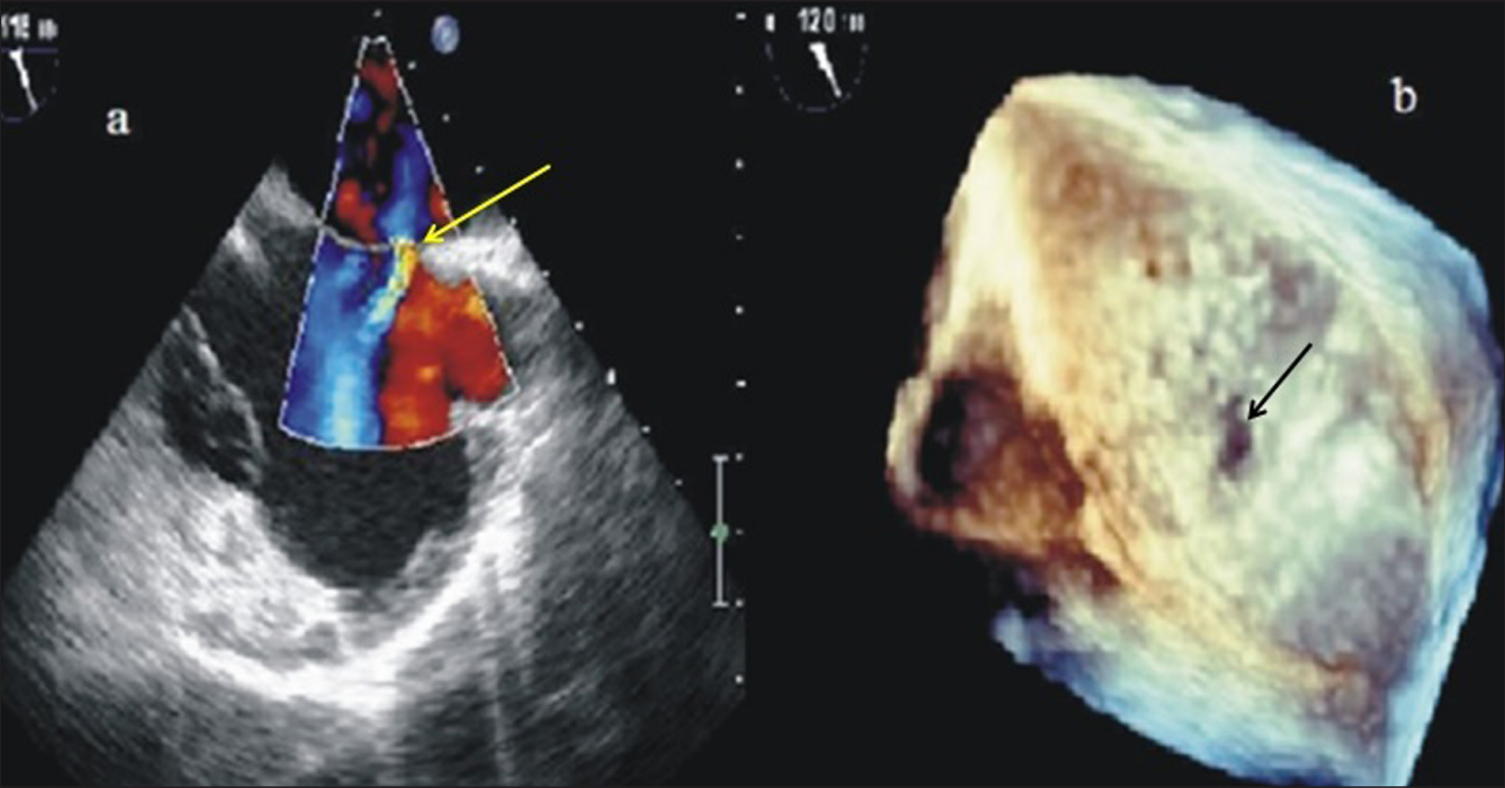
Echocardiography showing atrial septal defect image on color Doppler imaging on bicaval position (a) (yellow arrow) and on 3D imaging (b) (black arrow).
